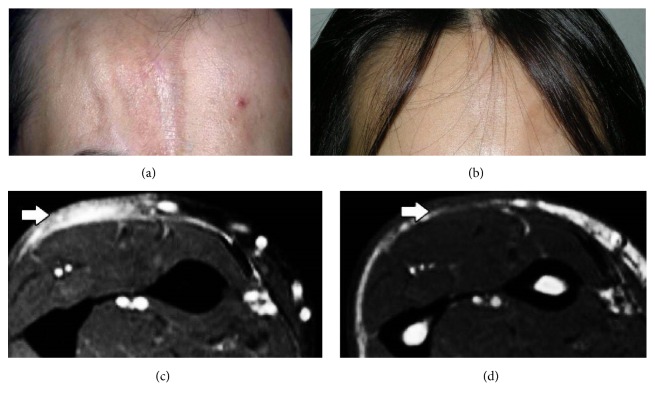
A patient with linear morphea before (a) and after (b) treatment. MRI results before (c) and after (d) treatment.
